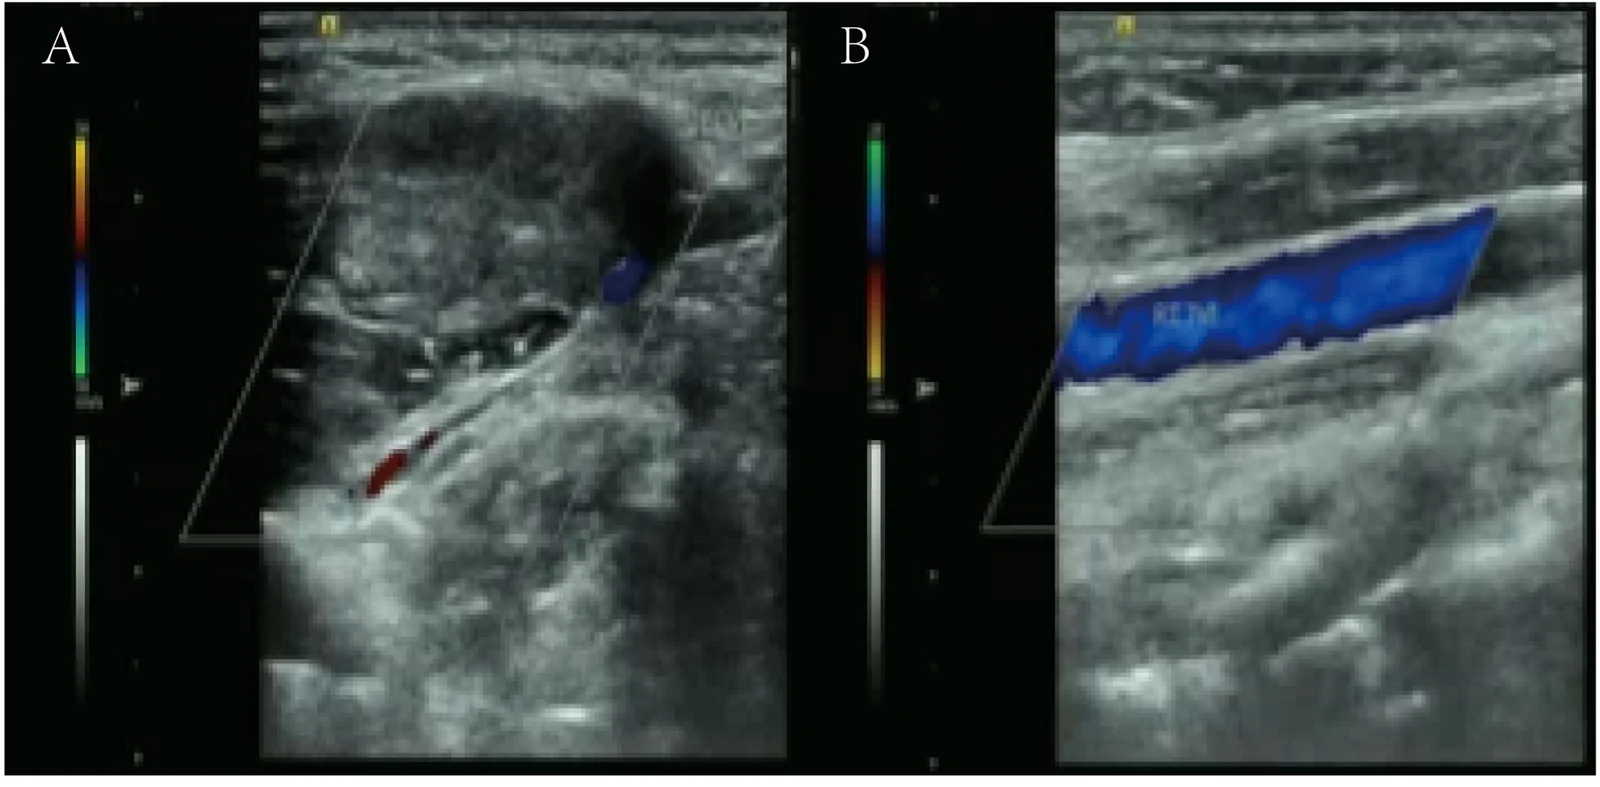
Pre-operative ultrasound imaging showing “cystic mass of the left neck”. (A) External jugular vein; (B) Internal jugular vein.
